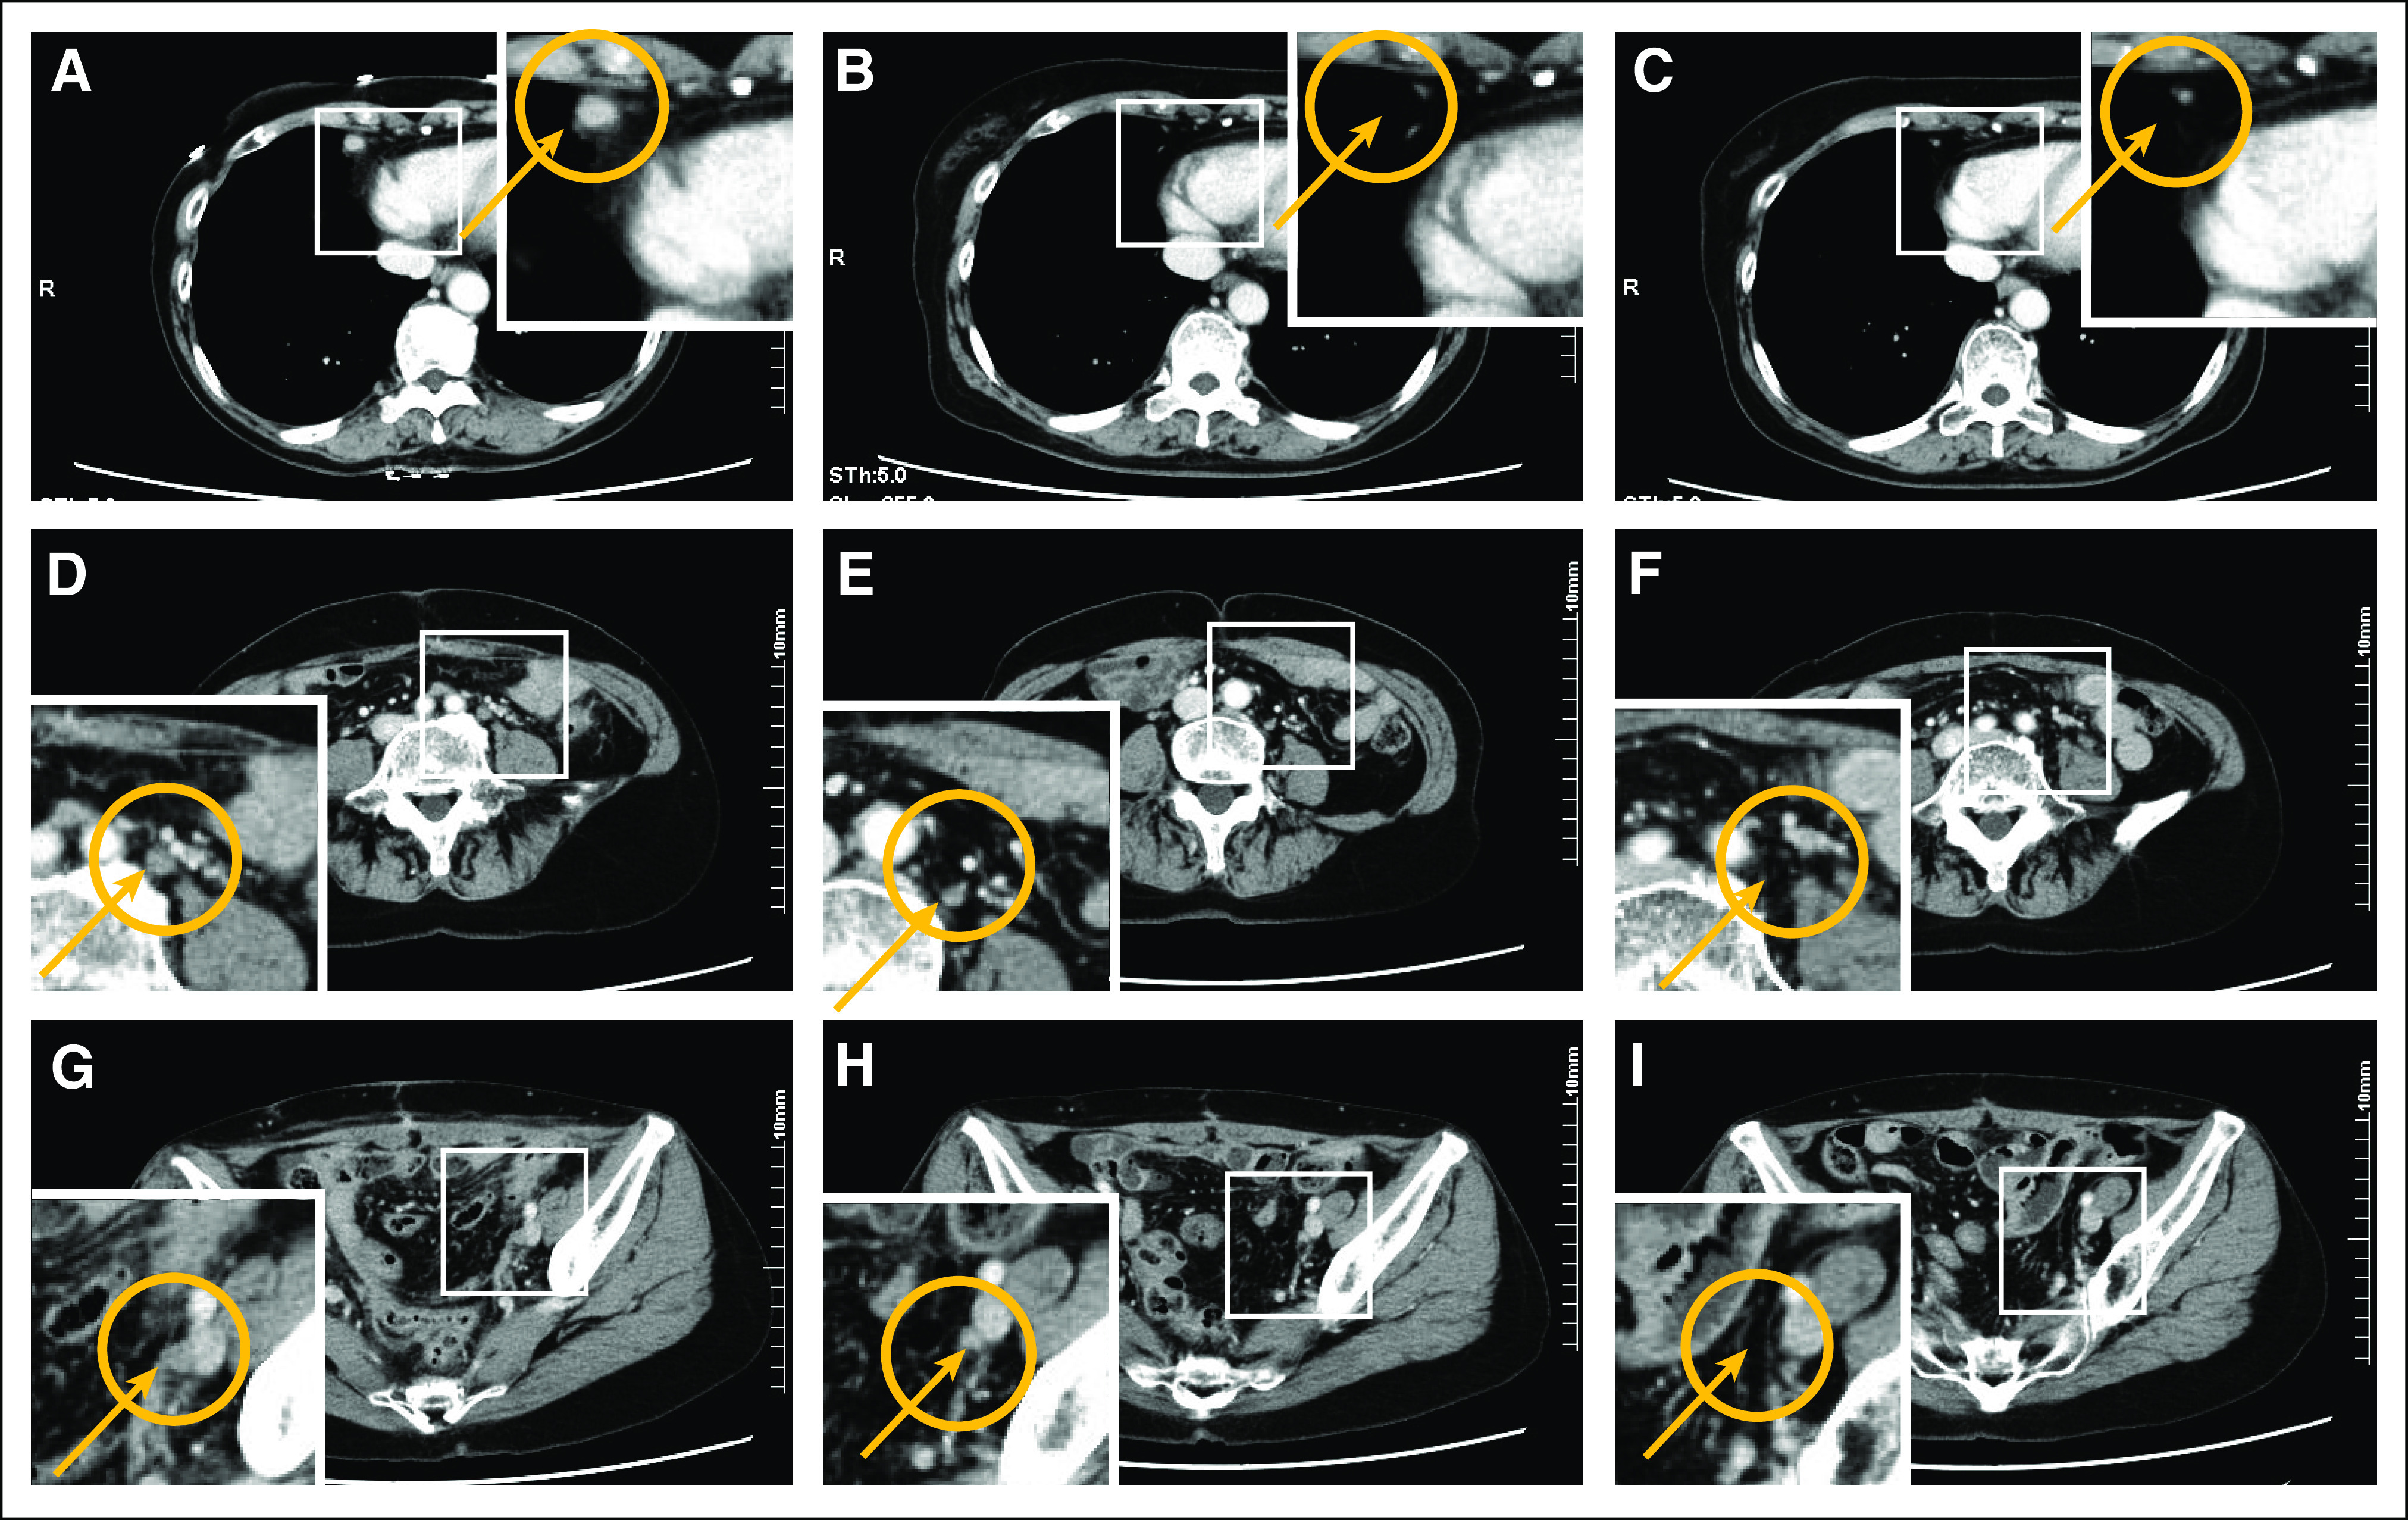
Shrinkage of the patient’s (A-C) cardiophrenic, (D-F) para-aortic, and (G-I) left lateral iliac lymph nodes after 3 months of olaparib treatment. Computed tomographic imaging of the abdomen and pelvis (A, D, G) before treatment, (B, E, H) after second-line chemotherapy, and (C, F, I) after olaparib treatment.
